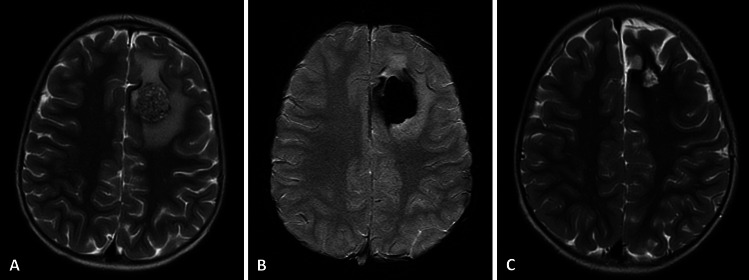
A 3-year-old male patient who presented with a first episode of seizures. Preoperative MRI A T2-weighted scan and B SWI scan showed CCM located in the left frontal lobe. The patient underwent gross total removal of the CCM. C Postoperative MRI T2-weighted scan confirmed gross total resection of the lesion
